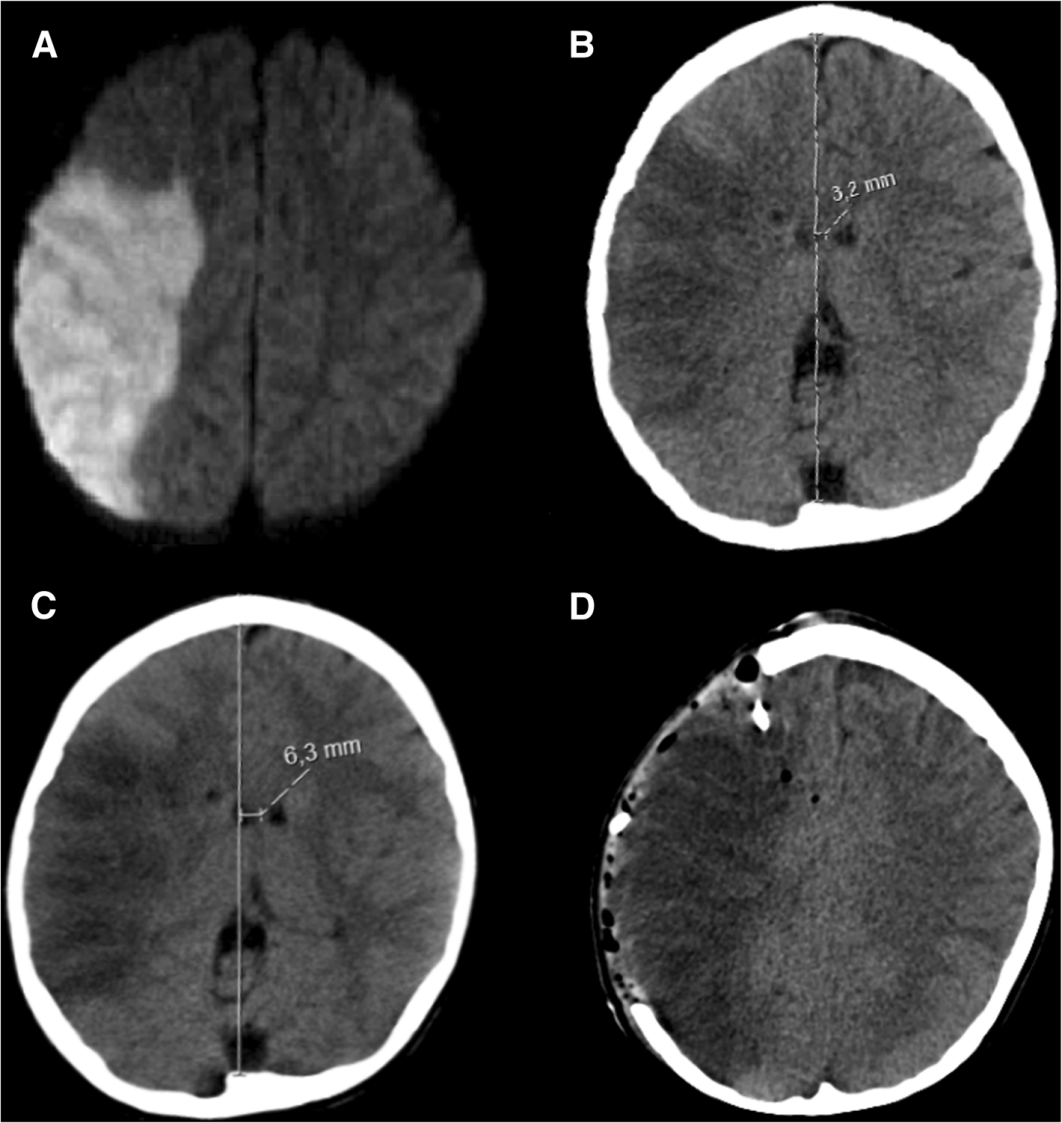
Pediatric ischemic stroke. A representative example of pediatric ischemic stroke in a 6-year-old boy with sickle cell anemia: axial diffusion-weighted MRI sequence (a) with increased signal in the right MCA territory, indicating ischemic infarction. Axial CT scan obtained on day 1 after stroke onset (b), showing a demarcated infarct with 3.2 mm midline shift. Axial CT scan obtained on day 2 after stroke onset (c), revealing a progressive midline shift of 6.3 mm, correlating with neurological deterioration. Axial CT scan after hemicraniectomy and implantation of a right frontal intraparenchymal ICP probe (d), with reversal of midline shift
